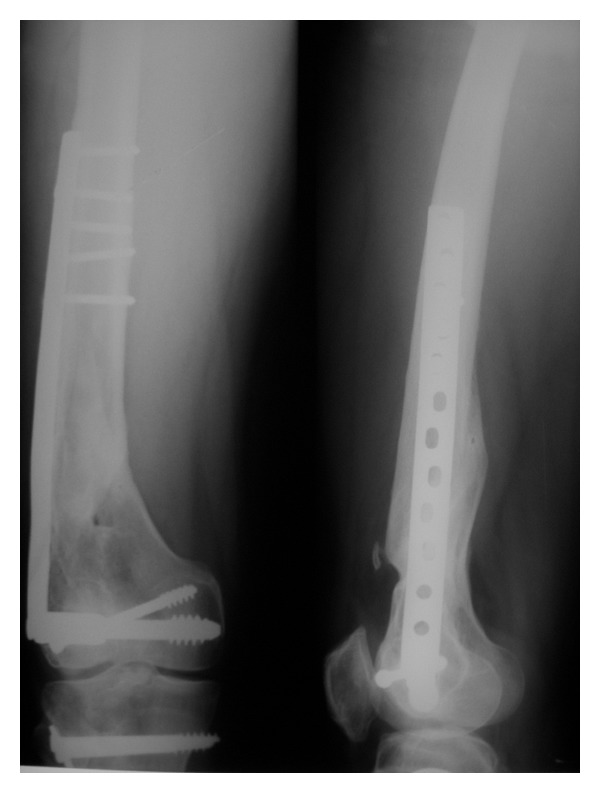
X-ray right femur AP and lateral views at 2-year followup showing fracture union.
